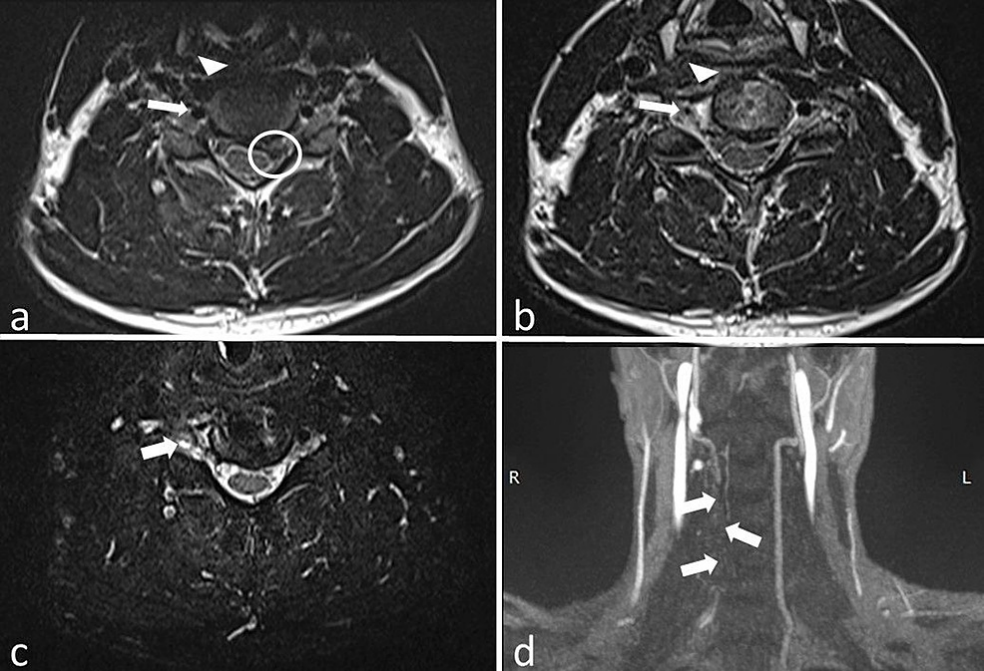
Cervical MR and MR Angiography Axial pre-(a) and post-(b) operative T2-weighted images demonstrate the change in the caliber of the right vertebral artery (arrows) along with the prominent atrophy in the right longus colli muscle (arrowheads). The preoperative extruded C5-6 intervertebral disk is also shown in the circle (a). Axial T2-space image (c) depicts a pseudomeningocele cavity at the post-foraminal segment of the right C5 nerve (arrow). Coronal MR angiography image (d) confirms the asymmetrical absence of flow in the right vertebral artery (arrows). MR, magnetic resonance.
